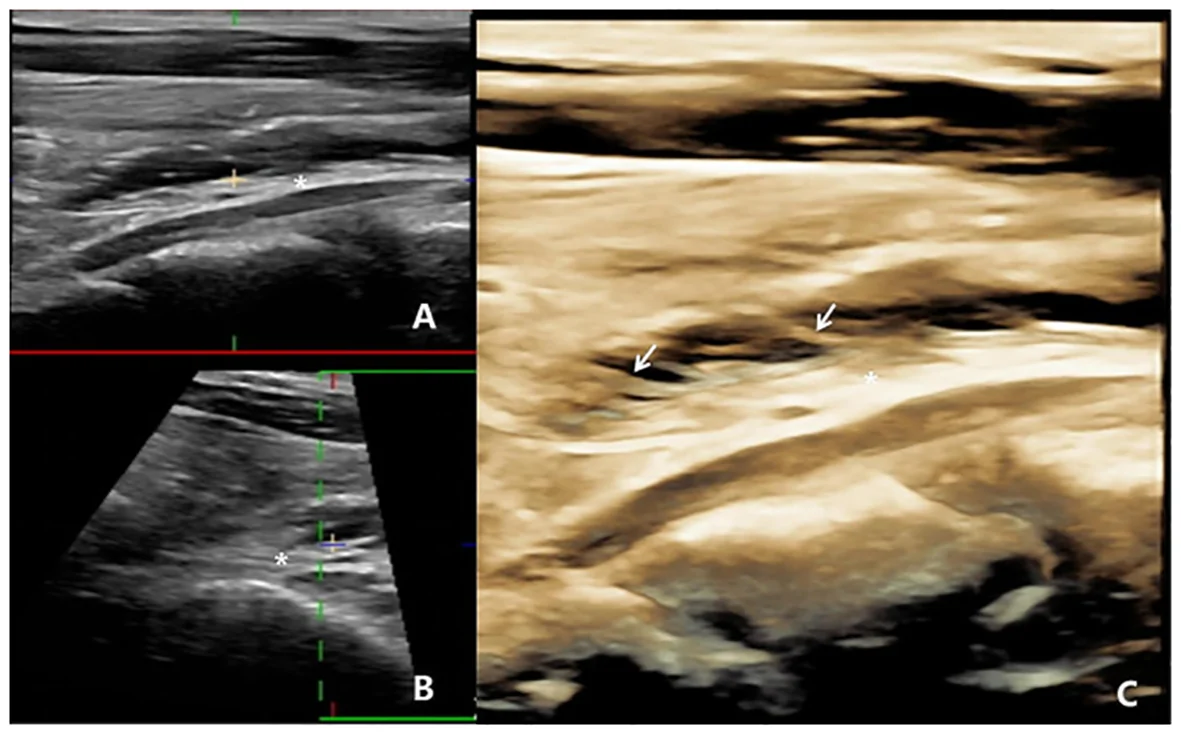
3D imaging of the recurrent laryngeal nerve (RLN) after water isolation. (A) Longitudinal view of the RLN (asterisk). (B) Transverse view of the RLN (asterisk). (C) 3D ultrasound imaging showing multiple small branches of the RLN (asterisk) (white arrows). Reproduced with permission (51).
